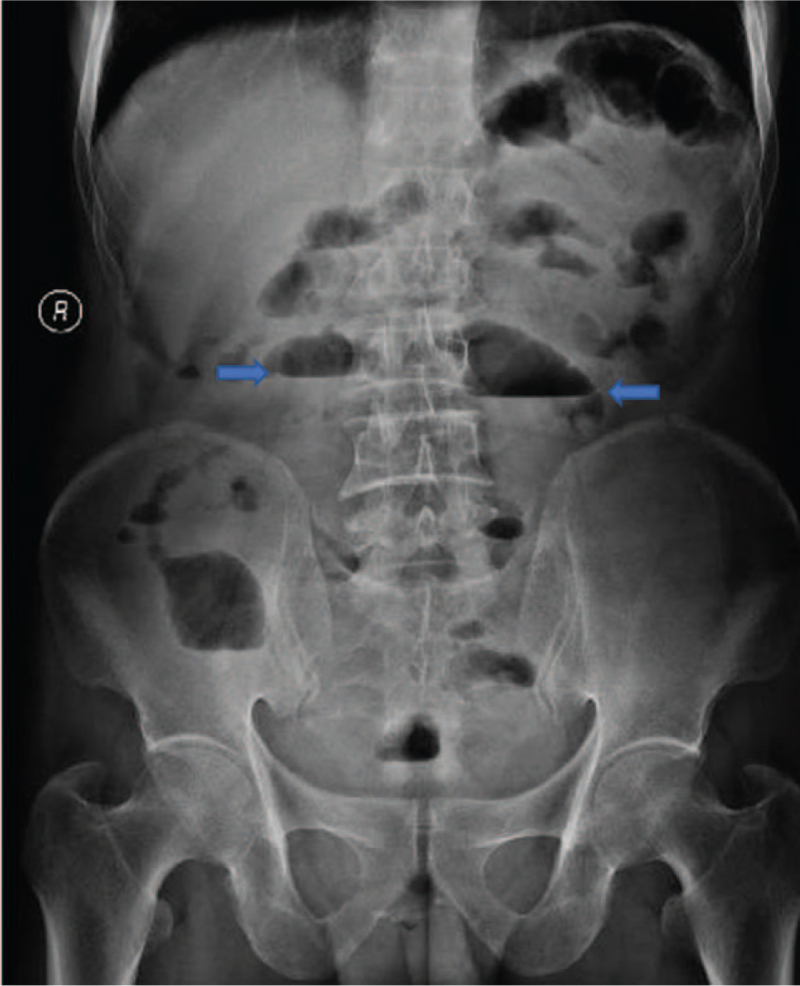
Abdominal X-ray showed an intestinal obstruction (arrows indicate the multiple gas–liquid planes).
